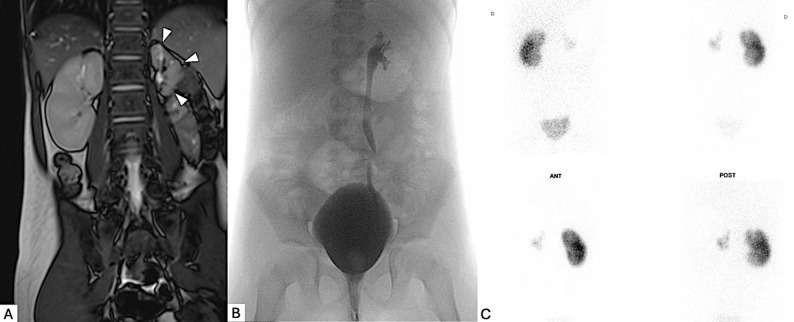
Multimodal imaging of unilateral renal hypoplasia with associated arterial stenosis. A: Coronal T2 MRI showing marked reduction in the size of the left kidney consistent with hypoplasia (white arrow), unlike the right kidney, which is of normal size. B: Cystography showing dilatation of the left ureter with reflux of contrast medium into the hypoplastic kidney, consistent with high-grade left vesicoureteral reflux. C: DMSA scintigraphy (anterior and posterior views) revealing decreased perfusion and tracer uptake in the left kidney compared to the right kidney
